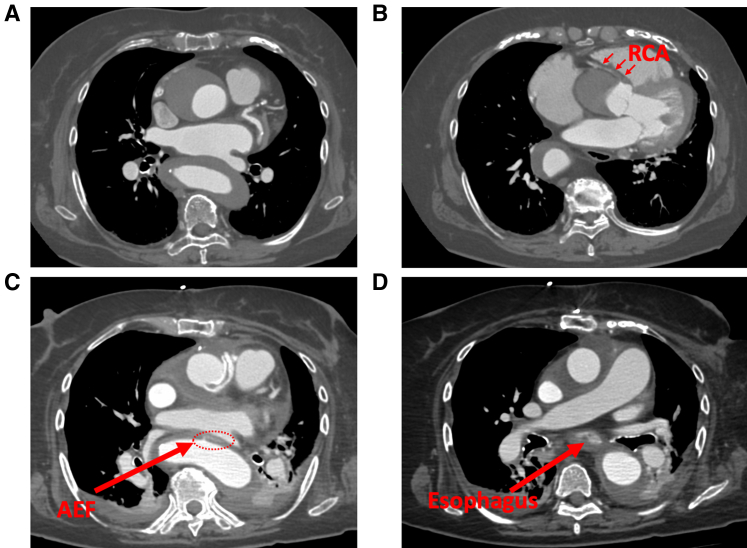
Preoperative and postoperative contrast-enhanced CT images. A and B, Preoperative CT images obtained at the onset of acute type A IMH. C and D, Postoperative CT images obtained at the onset of AEF. A, Circumferential thickening of the ascending aortic wall consistent with type A IMH. The ascending aortic diameter measured 45 mm with hematoma thickness of 20 mm. The descending aortic diameter at the future fistula site was 27 mm with hematoma thickness of 5 mm. B, Long-segment narrowing of the RCA near its origin, suggesting coronary involvement. C, Focal contrast extravasation from the descending thoracic aorta into the esophagus, consistent with AEF. At this level, the aortic diameter measured 25 mm with hematoma thickness of 2 mm, indicating continued remodeling without aneurysmal dilatation. The dashed circle indicates the esophagus at the compressed segment. D, Contrast pooling within the esophageal lumen proximal to the fistula, further supporting the diagnosis of AEF. *AEF*, Aortoesophageal fistula; *RCA*, right coronary artery.
